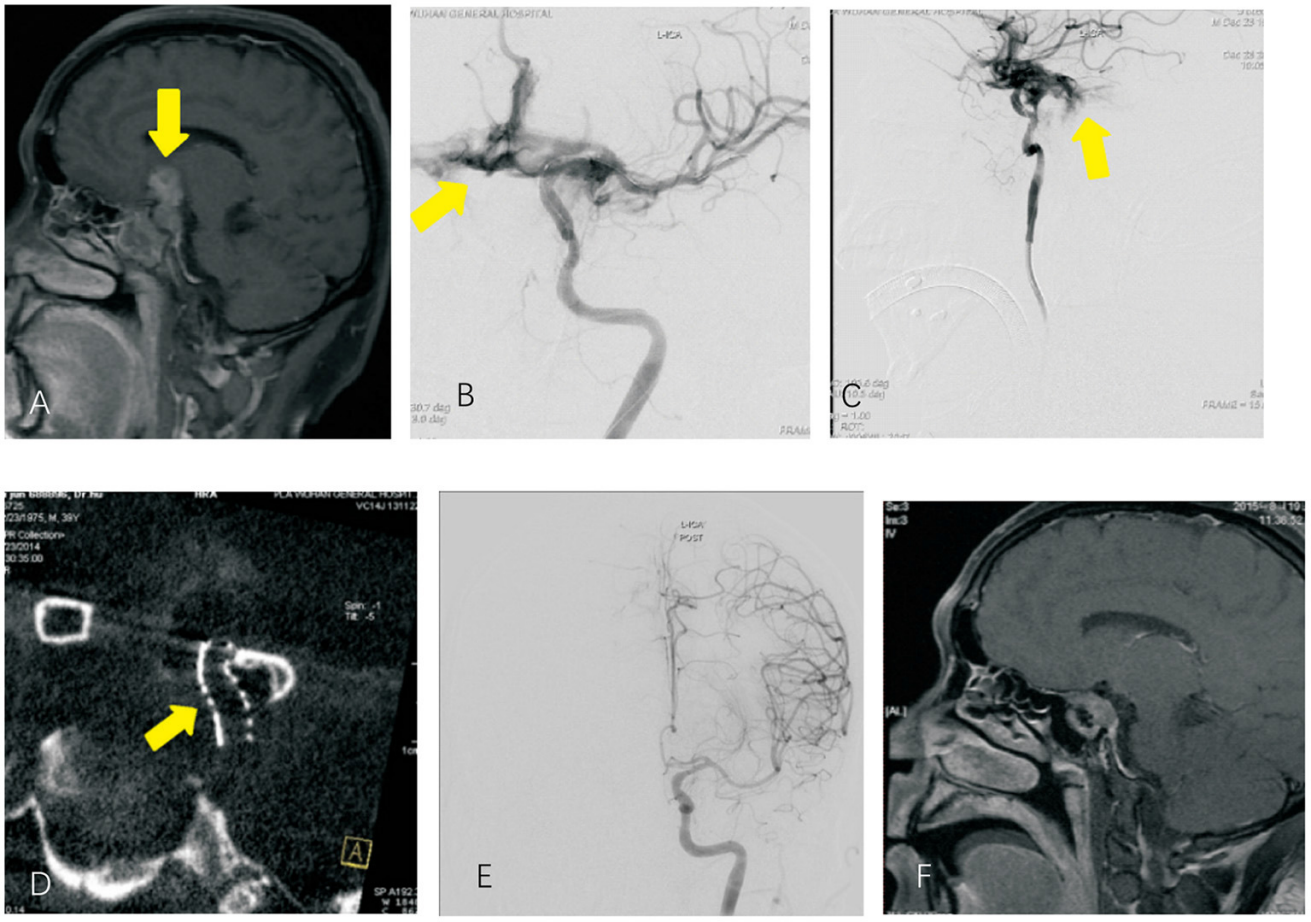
Invasive pituitary adenoma and intraoperative internal carotid artery rupture (A). Anterior MRI sagittal image, which showed pituitary adenoma; (B,C) DSA image of the ophthalmic segment of internal carotid artery rupture, which showed contrast agent spillage from the rupture to the arachnoid membrane; (D) maximum intensity projection showed good apposition of the Willis covered stent; (E) successful repair of the internal carotid artery after stent implantation; (F) postoperative MRI suggesting tumor resection.
